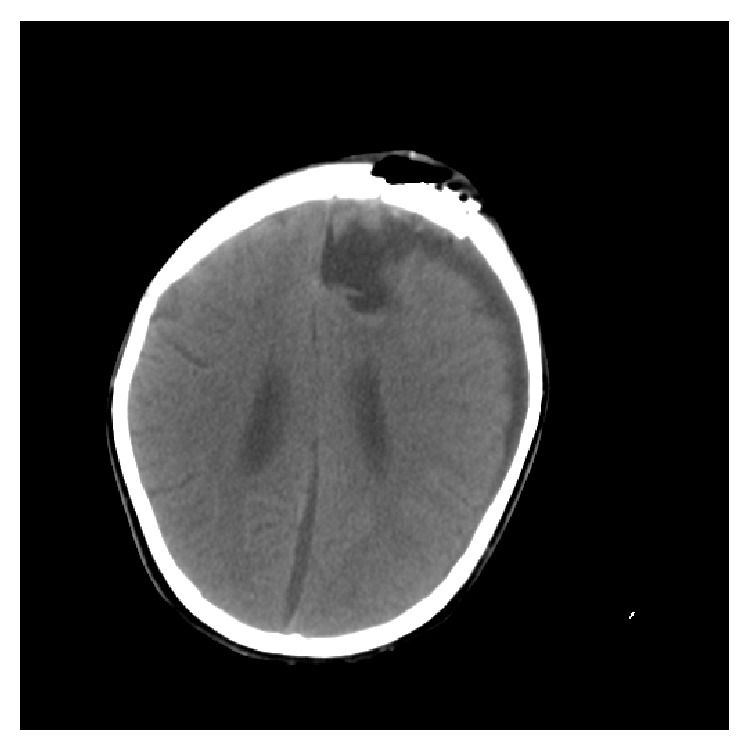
Primary brain CT scan that shows unilateral frontal brain abscess (confined to one hemisphere).
